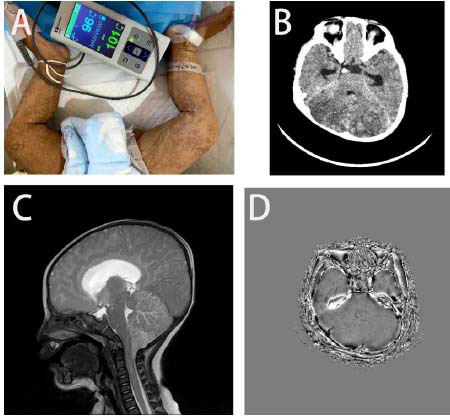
(A) Hyperpigmented whorls; (B) CT showing cerebellar calcifications; (C) T2- hyper intensities; (D) SWI micro hemorrhages.
